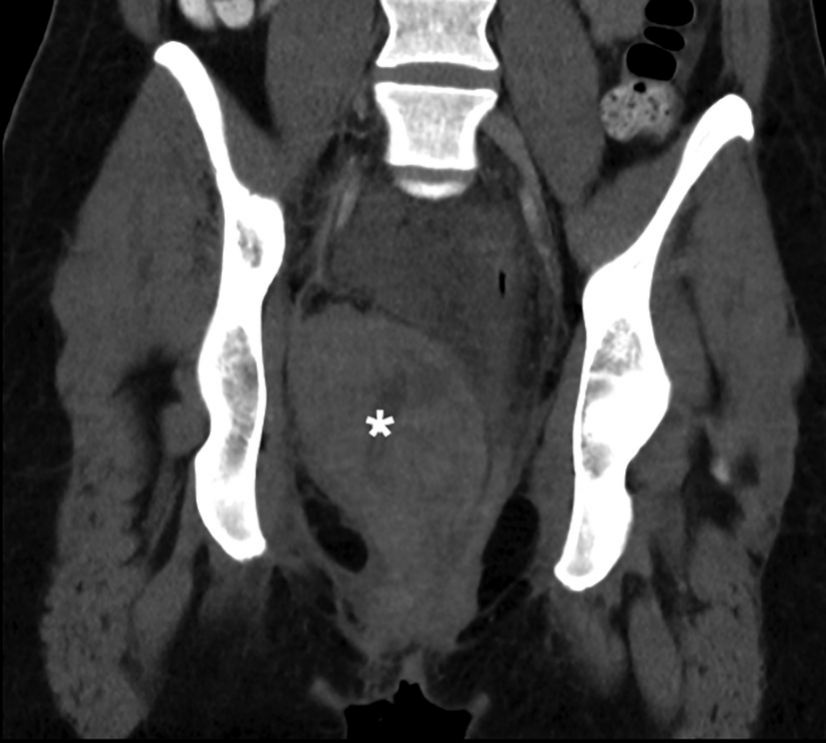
CT scan showing a large paravaginal haematoma extending to the supralevator space*.
